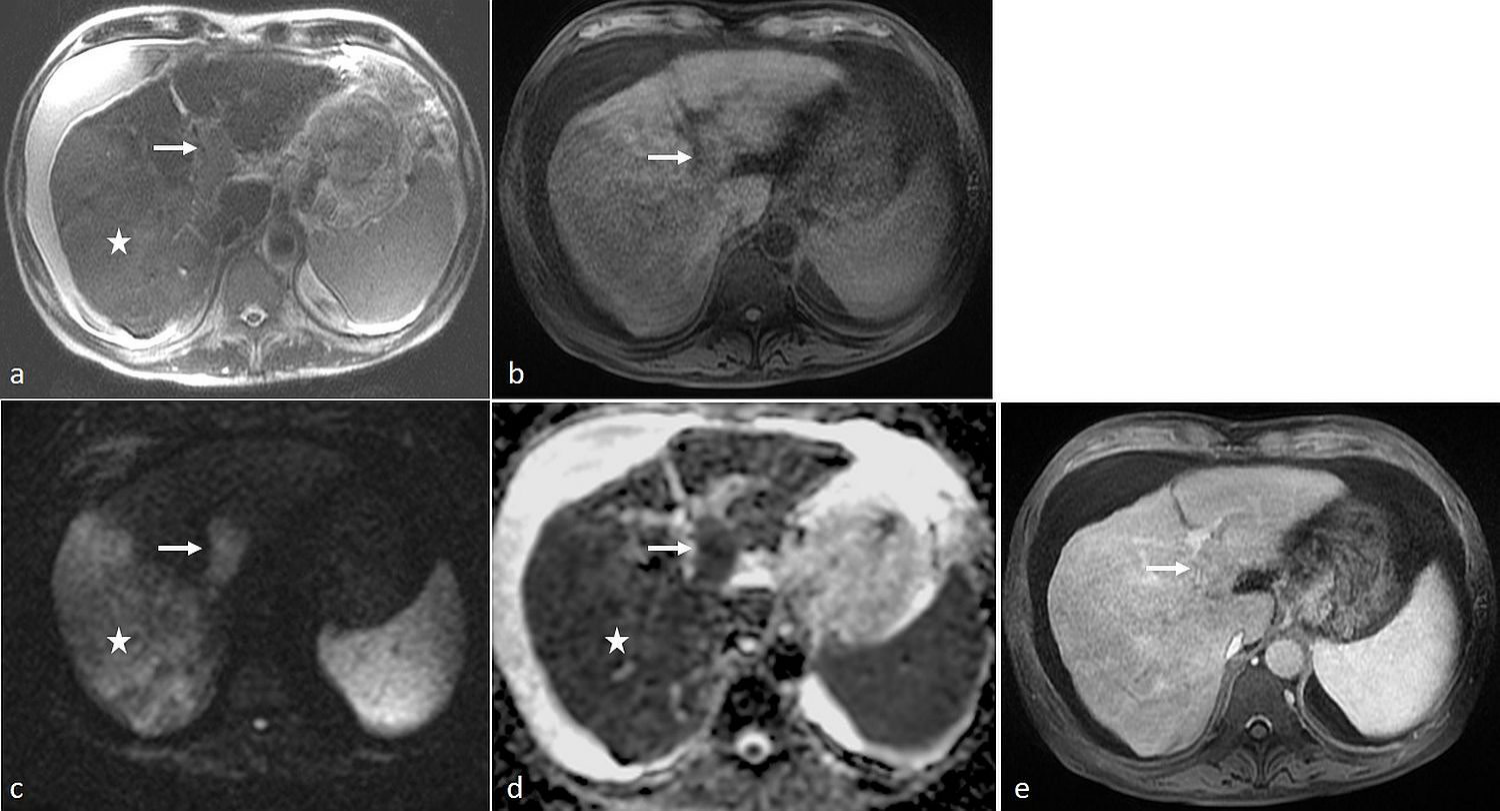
A 68-year-old gentleman presented with jaundice and abdominal distension. MRI was performed with intravenous administration of Dotarem. (a) On axial *T*
